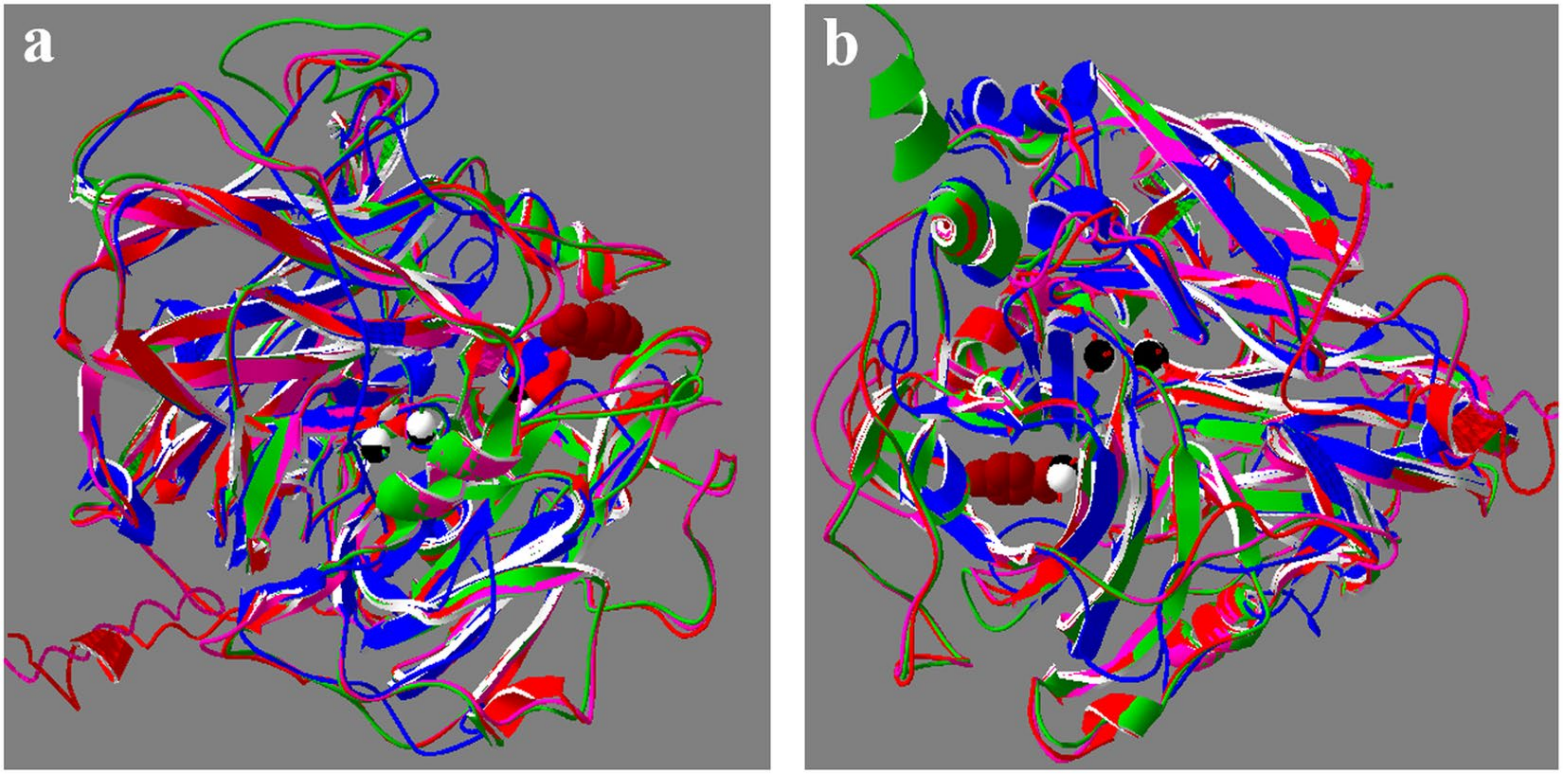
Models of fruit LMCOs superimposed on the X-ray structures of ascorbate oxidase (Ao) from *Cucurbita pepo* and laccase from *Trametes trogii* (Tt) and shown from two different perspectives (a,b). Red, cherry LMCO; pink, litchi LMCO; green, Ao; blue (Tt). Copper atoms from Ao (white) and Tt (black) are also superimposed. Substrate-binding pocket is depicted with brown space-filled substrate (2,6-dimethoxyphenol) and the copper atom nearest to it is T1. Blue/red space filled atoms showed His residue involved in abstracting electrons from the substrate and relying to the T1 Cu atom.
